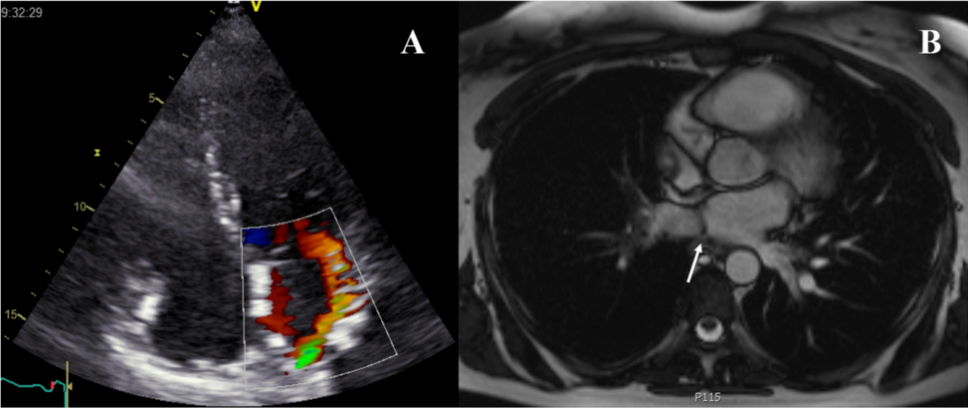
Transthoracic apical 4-chamber view with color Doppler (a) showing turbulence in the roof of the left atrium near the right pulmonary vein suggesting pulmonary venous obstruction or stenosis. On cardiac magnetic resonance imaging (b), a membrane (*arrow*) was demonstrated in the left atrium, with the left pulmonary veins draining into the left atrium, and the right pulmonary veins into the accessory (proximal) atrial chamber
